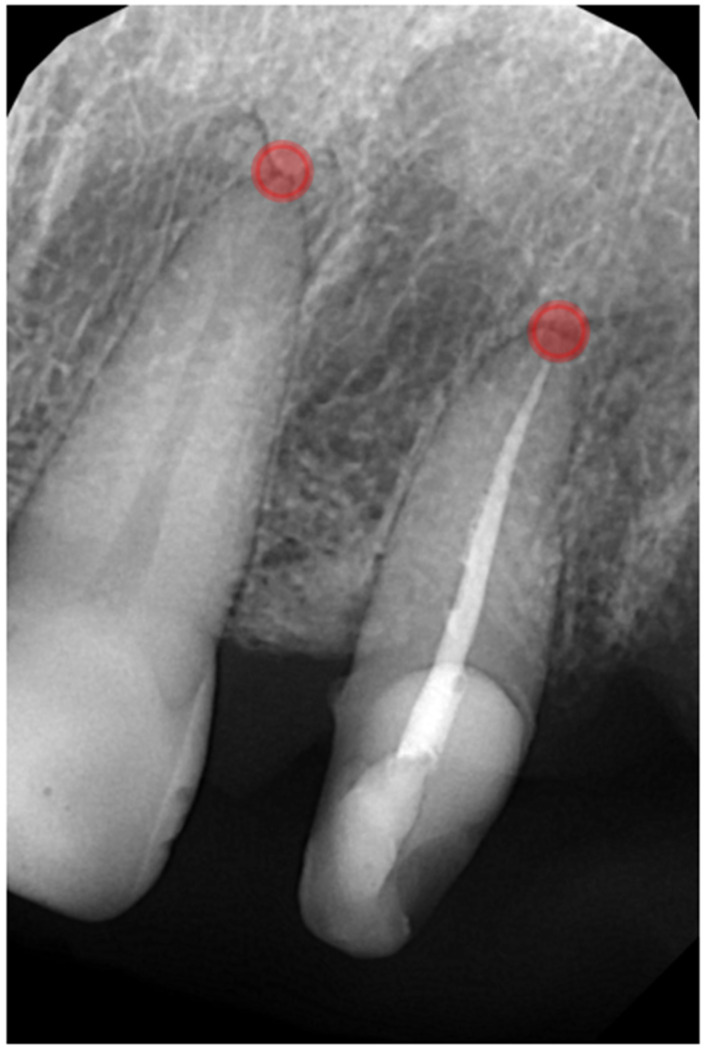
Example periapical radiograph with overlaid circular regions of interest used for radiomic analysis. All ROIs are displayed with a fixed radius of 40 pixels, corresponding to the sampling area used for radiomic feature extraction.
